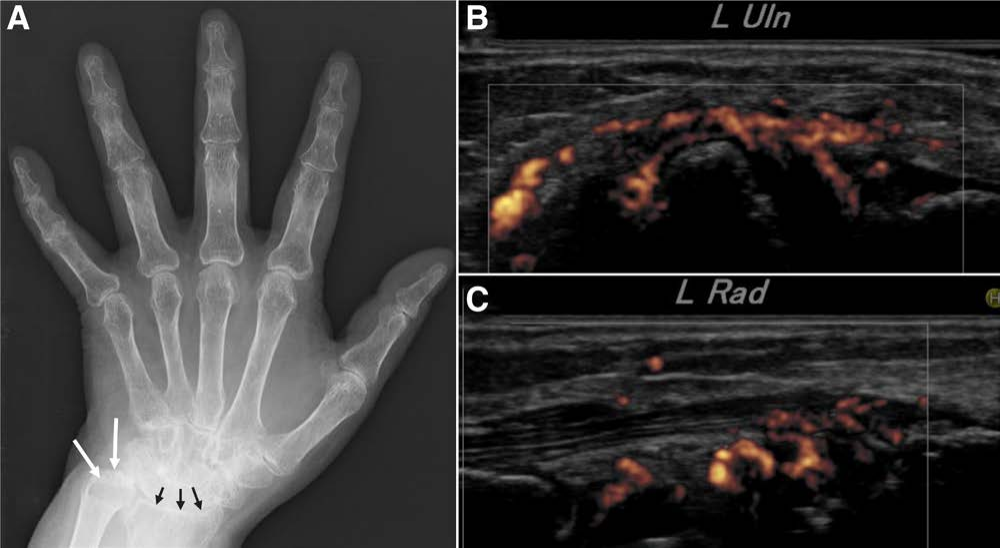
X-ray and US findings of the left hand. (A) X-ray of the left hand shows narrowing of the wrist joint (black arrows) and destruction of the ulnar styloid process (white arrows). Peripheral ulnar deviation from the metacarpophalangeal joint at 5th finger. Narrowing of the distal interphalangeal joints due to osteoarthritis was observed. US showed inflammation of the wrist joint on the (B) ulnar and (C) radial side. US = ultrasonography.
